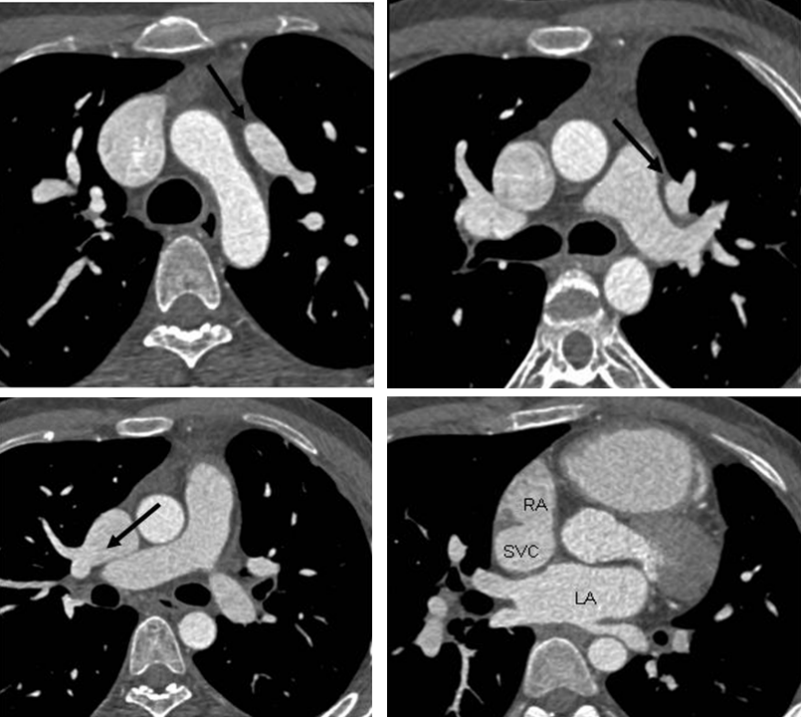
Partial anomalous pulmonary venous return of both lungs. An asymptomatic male patient with a dilated right side of the heart documented with thorax x-ray. The left upper lobe vein drains into the brachiocephalic vein (a-b). The right upper lobe vein drains into the superior vena cava (c). Veins of both lower lobes drain normally into the left atrium (d). RA = right atrium, LA = left atrium, SVC = superior vena cava.
